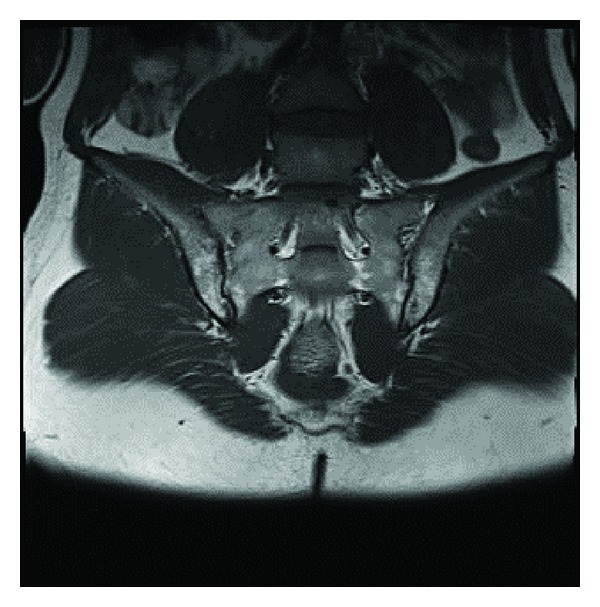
MRI of the sacroiliac joints revealed bilateral sacroiliitis.
